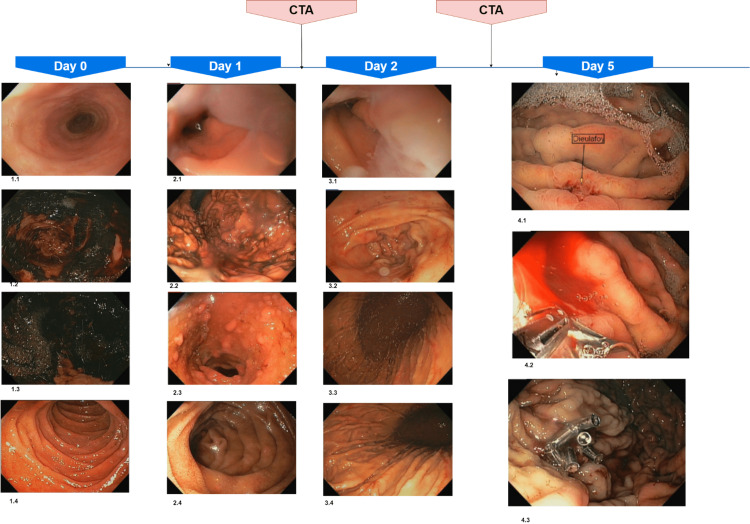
Timeline and number of upper endoscopic procedures required to identify the Dieulafoy lesion. CTA was performed between day 1 and 2, and day 4. 1.1 – esophagus, 1.2 – gastric antrum, 1.3 – gastric fundus, 1.4 –Second part of the duodenum 2.1 – esophagus, 2.2 – gastric fundus, 2.3 – duodenal bulb, 2.4 Second part of – duodenum 3.1 -  GE junction 3.2 – gastric antrum, 3.3 – gastric fundus, 3.4 – gastric body 4.1 – Dieulafoy in mid-gastric body, 4.2 – bleeding Dieulafoy vessel, 4.3 – status post local epinephrine and endoclips CTA, CT angiography
